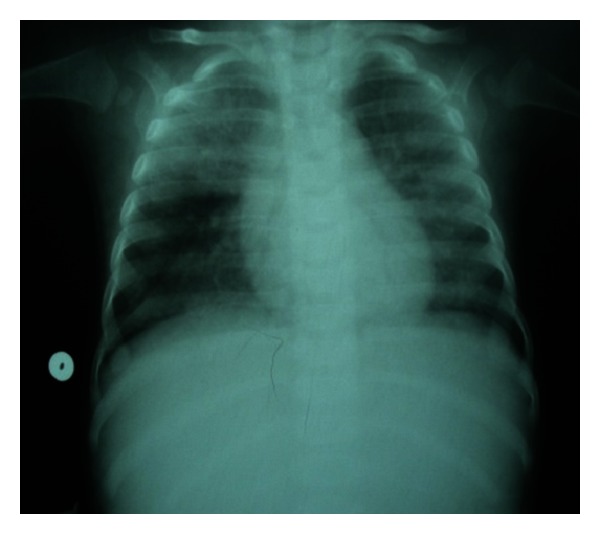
Chest X-ray: bilateral alveolointerstitial infiltrate.
